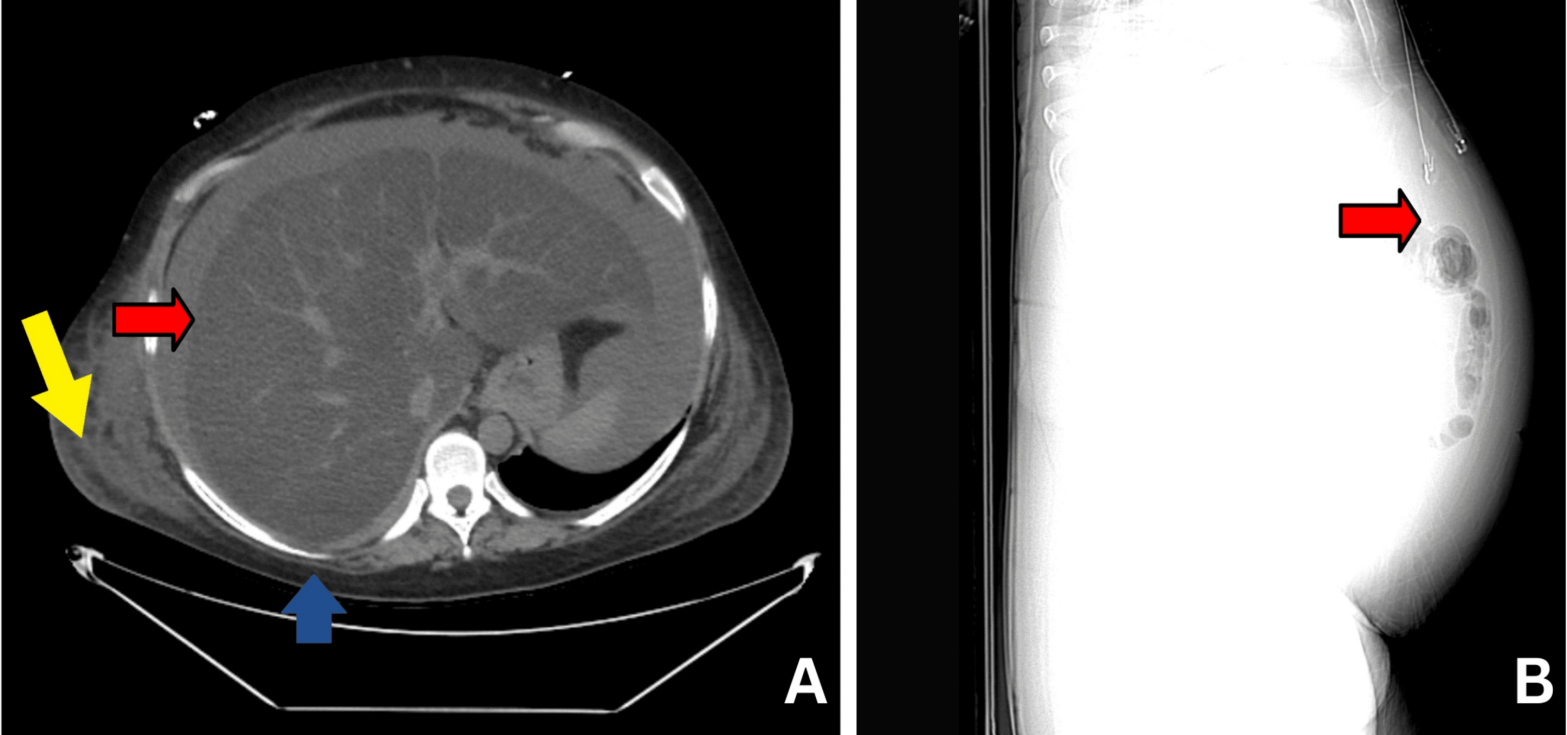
Day 1 imaging of WD hepatic manifestations (A) Day 1 ED non-contrast CT abdomen pelvis shows moderate ascites (red arrow), fatty liver, no free air or abscess, mild diffuse anasarca (yellow arrow), and atelectasis (blue arrow) in the inferior right lobe. (B) Day 1 CT abdomen pelvis in lateral view showing abdominal shadow representing ascites. WD: Wilson’s disease, ED: emergency department, CT: computed tomography
